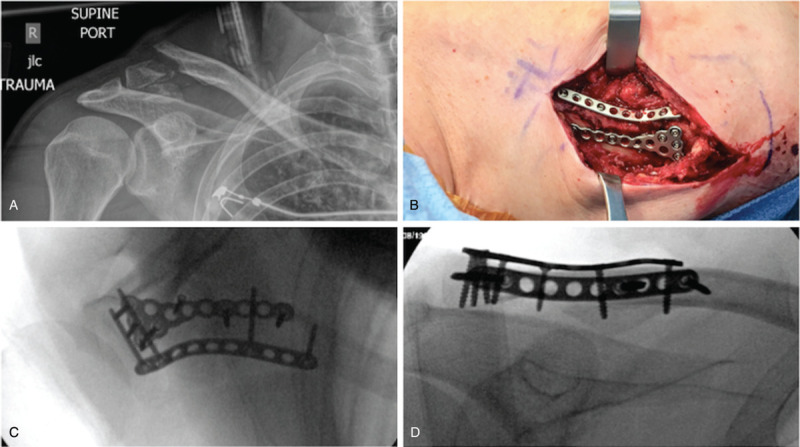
(A) Injury radiographs of another Neer type-V distal clavicle fracture. (B) An intraoperative photograph showing definitive fixation with a Y-shaped mini-fragment plate on the superior distal clavicle surface, and a straight mini-fragment plate contoured to the anterior distal clavicle surface. (C, D) Final fluoroscopic images demonstrating anatomic reduction and the dual mini-fragment plated construct with interdigitating locking screws in the distal fragment. A single lag screw prior to plate application was placed into the inferior fragment attached to the coracoclavicular ligaments.
